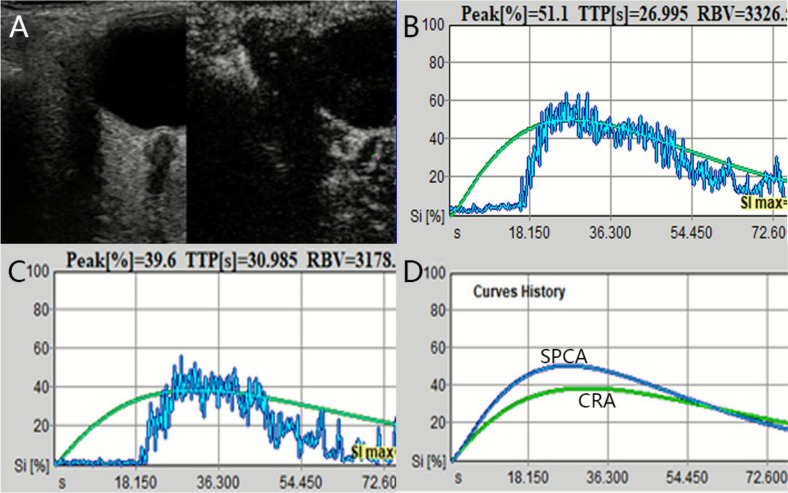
A CEUS image of a patient with severe intracranial hypertension. B The quantitative time-intensity curve analysis of SPCA, with the blue curve representing the actual SPCA measurement and the green curve showing its fitting curve. C The quantitative time-intensity curve analysis of CRA, with the blue curve representing the actual CRA measurement and the green curve showing its fitting curve. D The blue curve depicts the fitted SPCA analysis while the green curve represents the fitted CRA analysis. Abbreviations: *CEUS* contrast-enhanced ultrasound, *CRA* central retinal artery, *SPCA* short posterior ciliary artery
